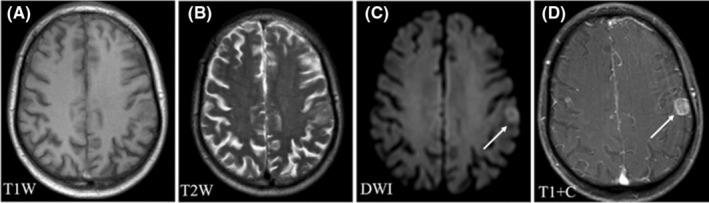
Pattern IV of brain metastases in SCLC. Pattern IV: A 78‐year‐old man was diagnosed with BMs of SCLC. The patient had numerous lesions located in the supratentorial and infratentorial areas. One lesion (white arrow) was located in the frontal lobe, with hypointensity in T1WI (A), hyperintensity in T2WI and DWI (B, C), and homogeneous enhancement (D). BMs, brain metastases; DWI, diffusion‐weighted imaging; SCLC, small cell lung cancer.
